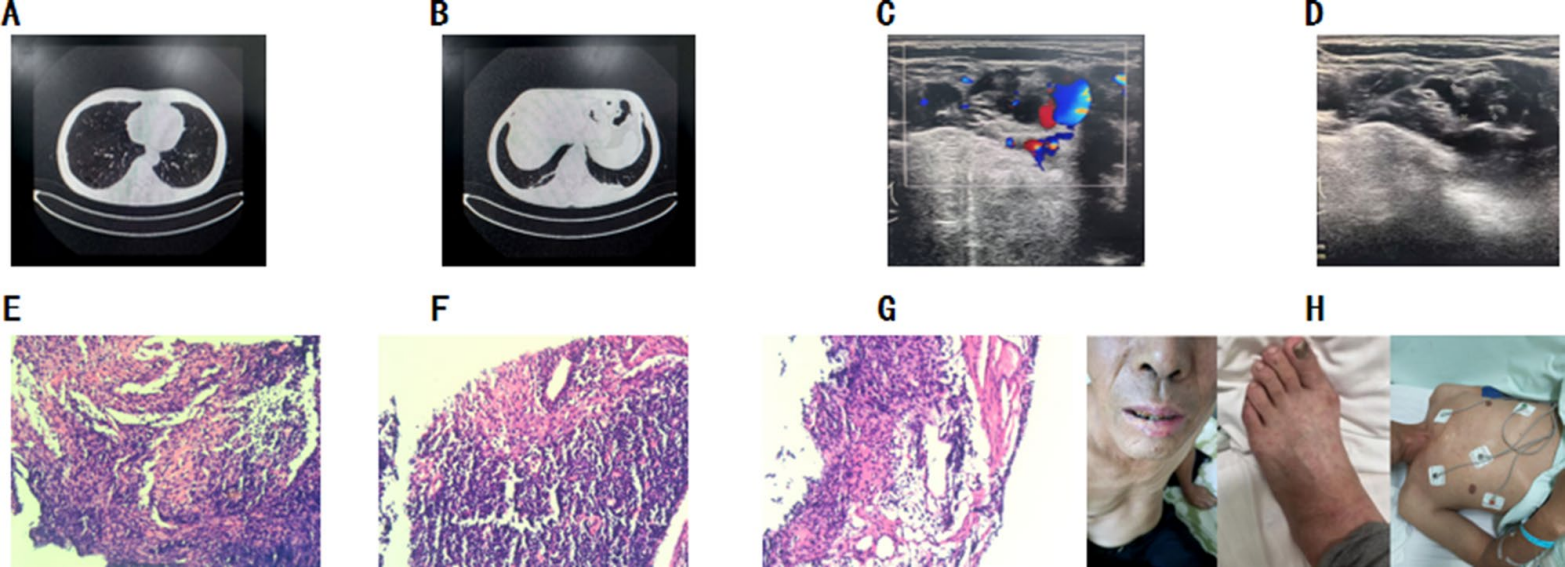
Relevant examination results. (A, B) Head, chest, and abdominal CT findings; (C–G) Neck lymph node ultrasound and pathological biopsy results; (H) The appearance of suspicious oral plaques during the patient’s treatment
